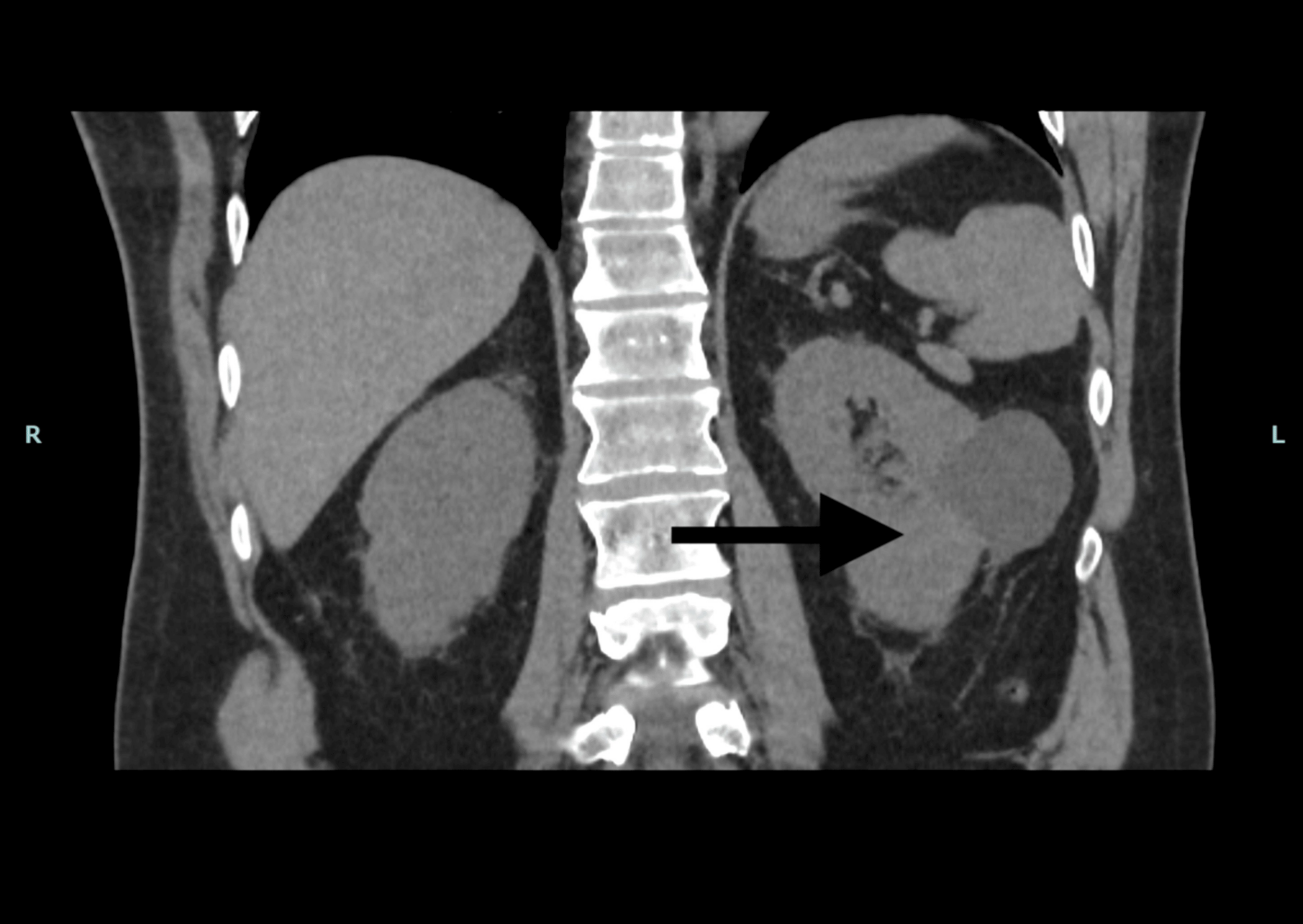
CT kidney showing an indeterminate left renal lesion with features suggestive of a haemorrhagic cyst. No enhancing or infiltrative characteristics were observed, supporting a benign process.
